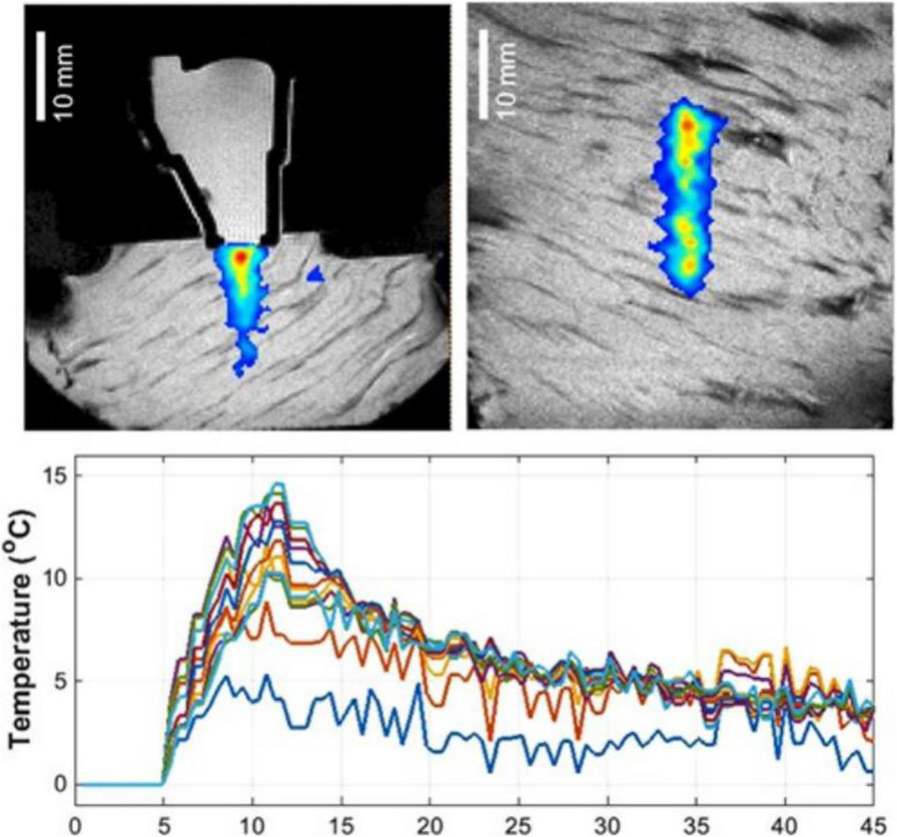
MRI image of applicator in pork muscle (axial section) after 7s showing thermal profile, and calculated temperature rise
